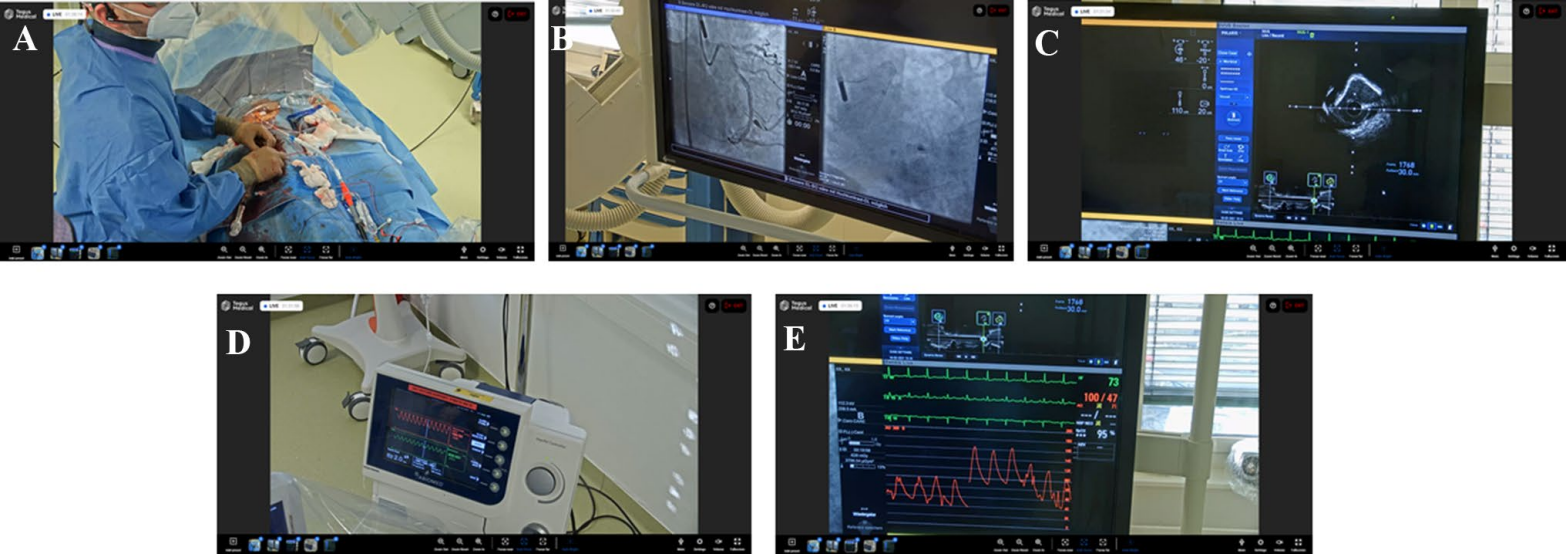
Screenshots from the TEGUS online platform of the operating field (A), angiography (B), intravascular ultrasound (C), the Impella^®^ controller (D), and hemodynamics (E)
